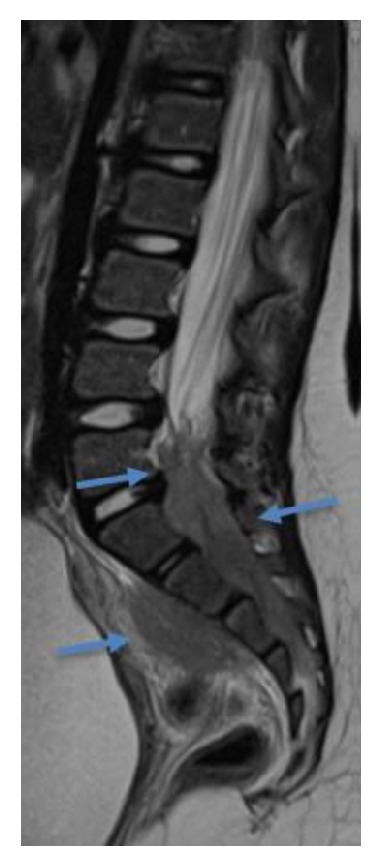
Sagittal T2-weighted MRI of the lumbosacral spine shows a large intracanalar epidural hypointense mass at L5 to S4, extending into the presacral space (blue arrows).
